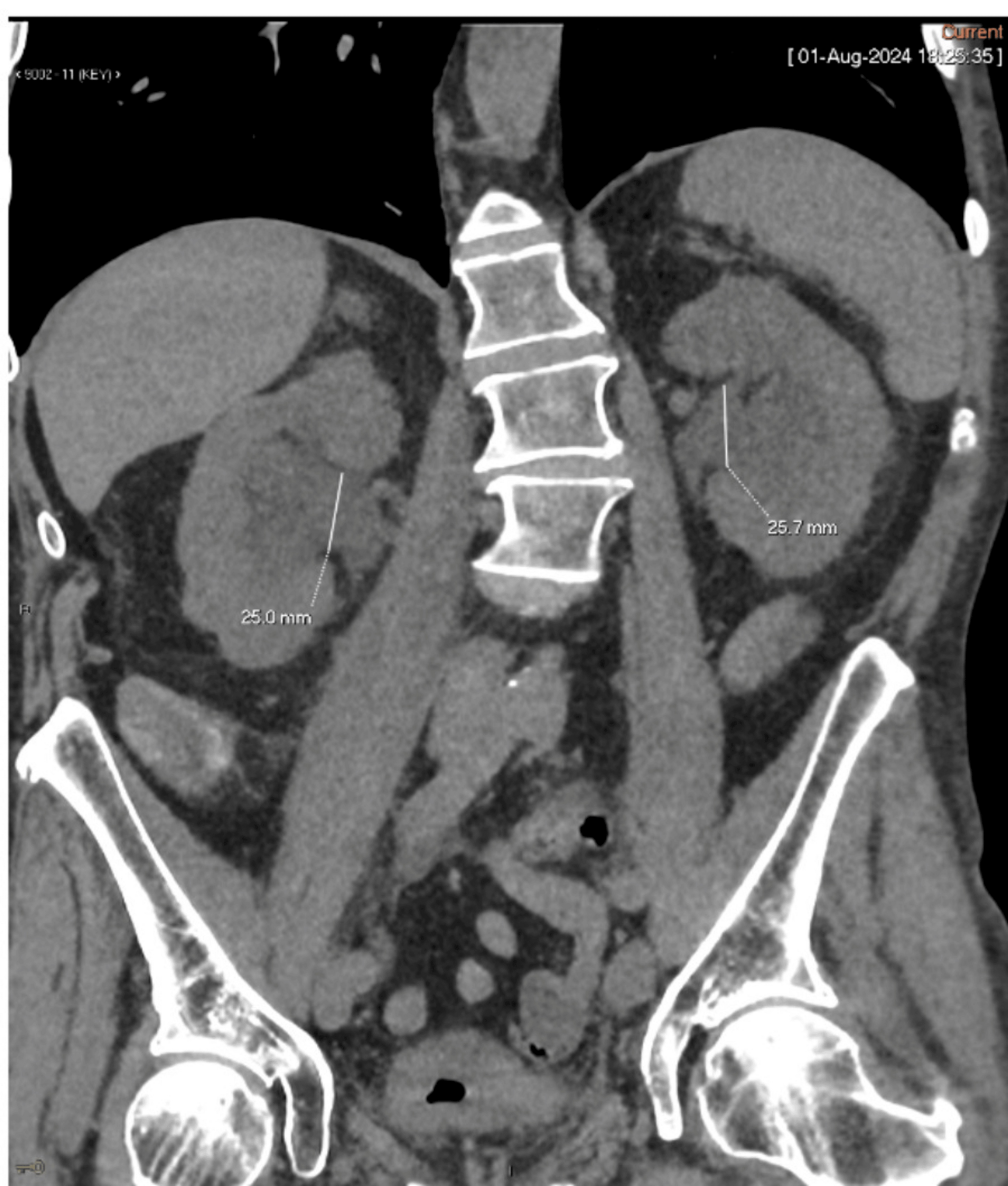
Coronal view of the CT scan of the abdomen and pelvis showing bilateral hydronephrosis
